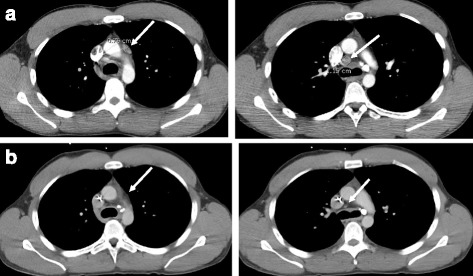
Response to PD-1 inhibition in patient with renal medullary carcinoma. a CT chest with contrast showing mediastinal lymphadenopathy prior to treatment with nivolumab (b) CT chest with contrast showing resolution of mediastinal lymphadenopathy after nine months on treatment with nivolumab
